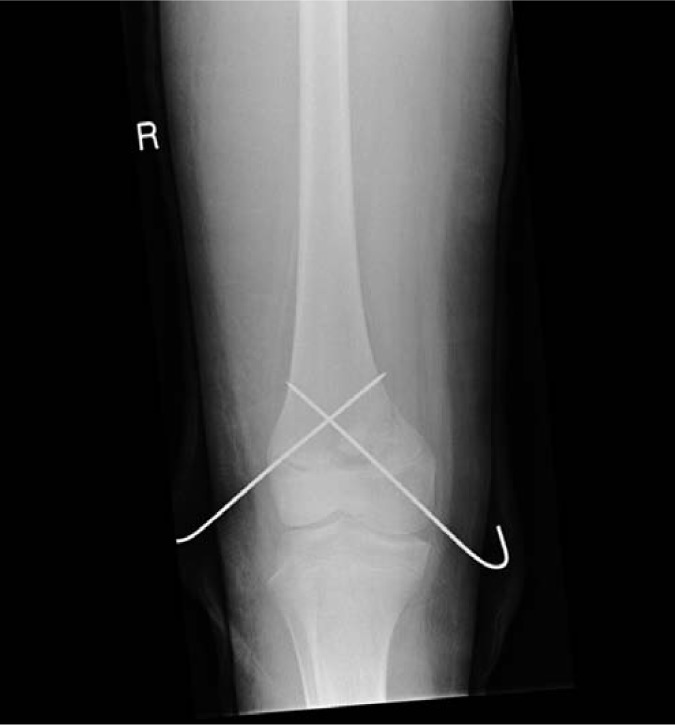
Postoperative AP radiograph showing reduction and Kirschner-wire fixation.
